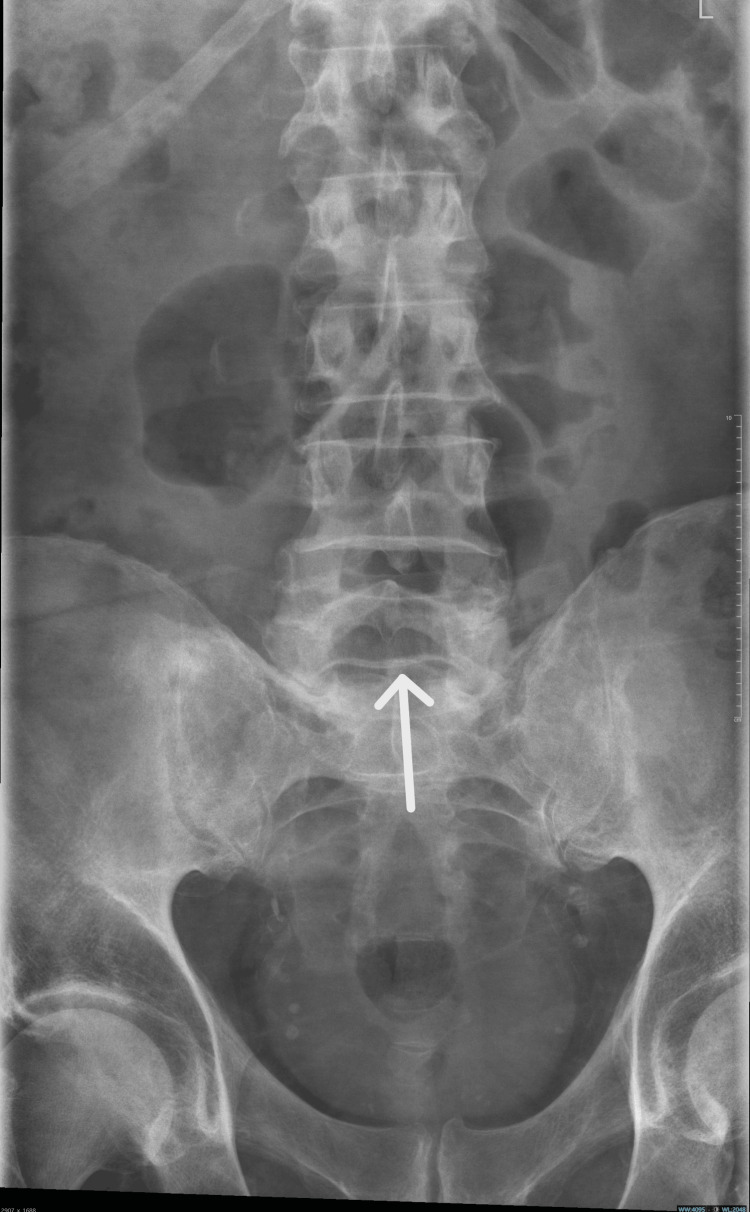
Anteroposterior lumbar spine X-ray showing minor degenerative changes at the lower lumbar levels (arrow), with preserved vertebral alignment
